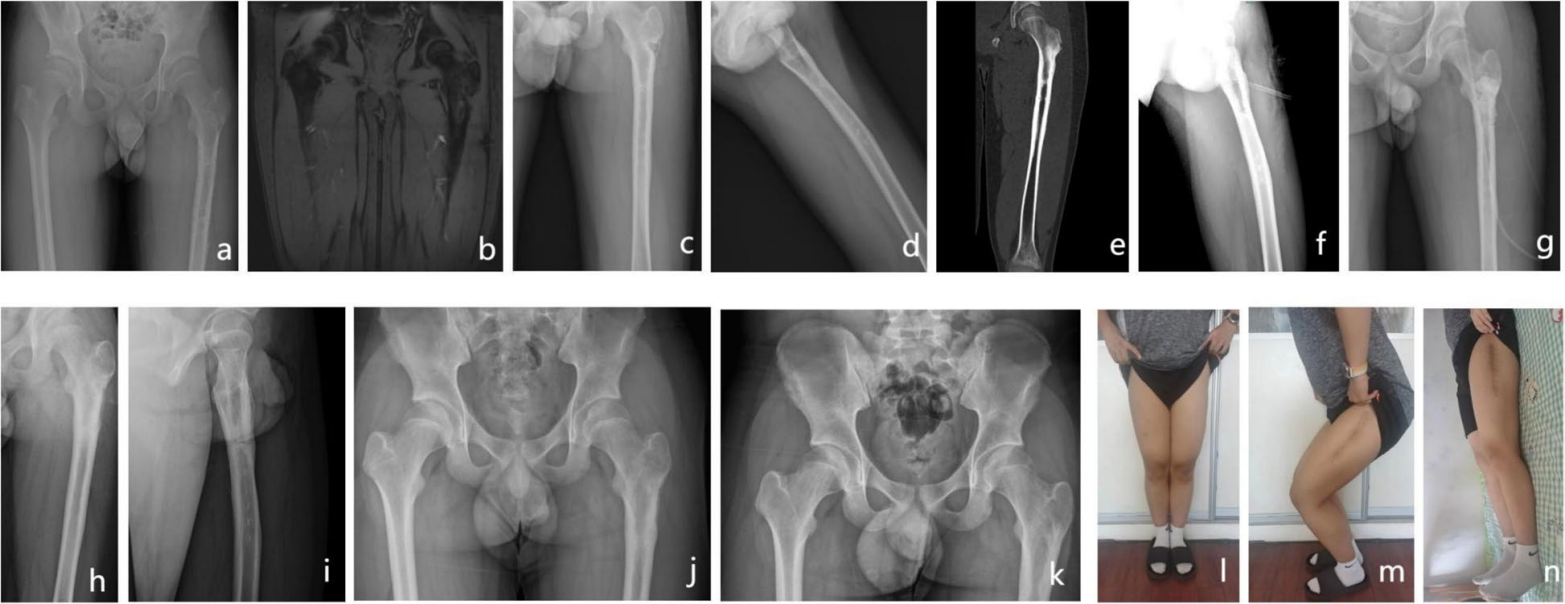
Patient, male, 15 years old, presented with osteomyelitis of the left femur with no obvious causative factors. a-e preoperative X-ray and CT examination showed infection of the left femur; f-g, h-i radical debridement and drug-laden calcium sulfate implantation showed anastomosis of the drug-laden calcium sulfate to the bone defect site on X-ray 1 and 3 days after surgery; j 6-month postoperative X-ray examination showed good healing of the bone defect site; k-n Post-operative X-ray at 36 months shows good post-operative recovery with full weight bearing of the affected limb and generally normal gait
